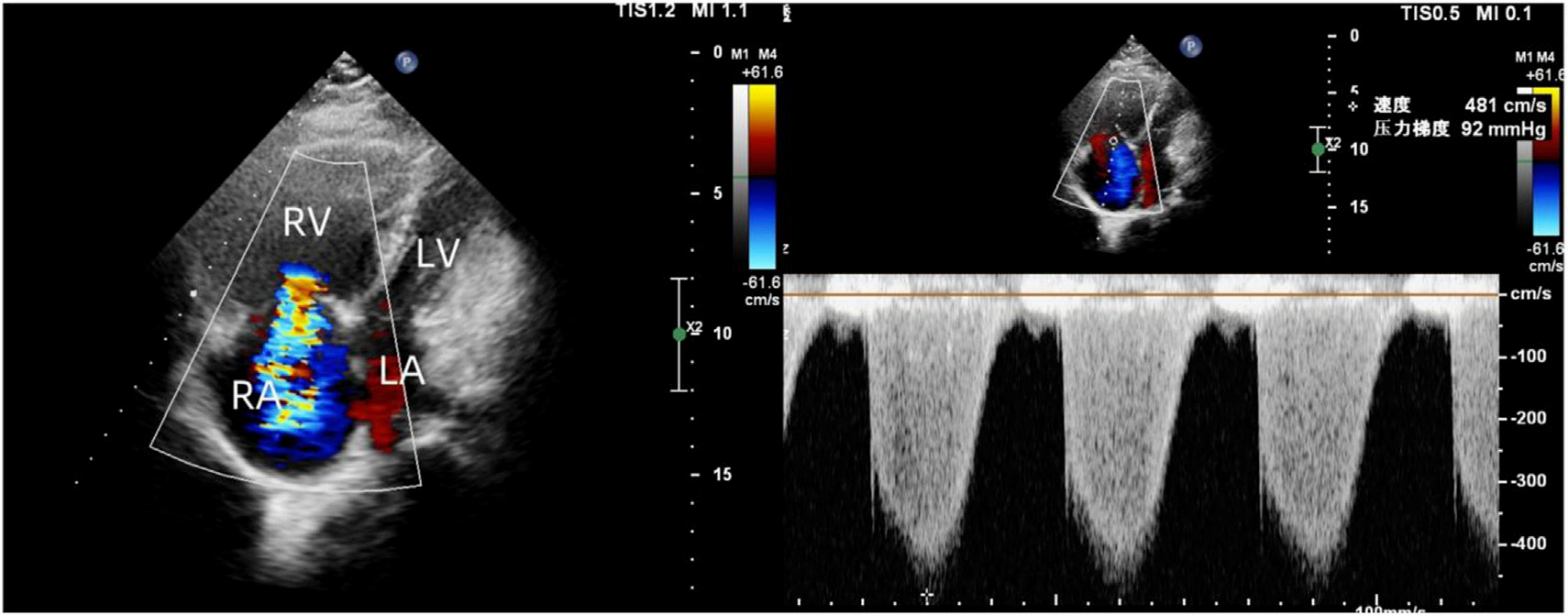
Transthoracic echocardiography revealed enlarged right heart, reduced right ventricular wall motion, and severe tricuspid regurgitation.
